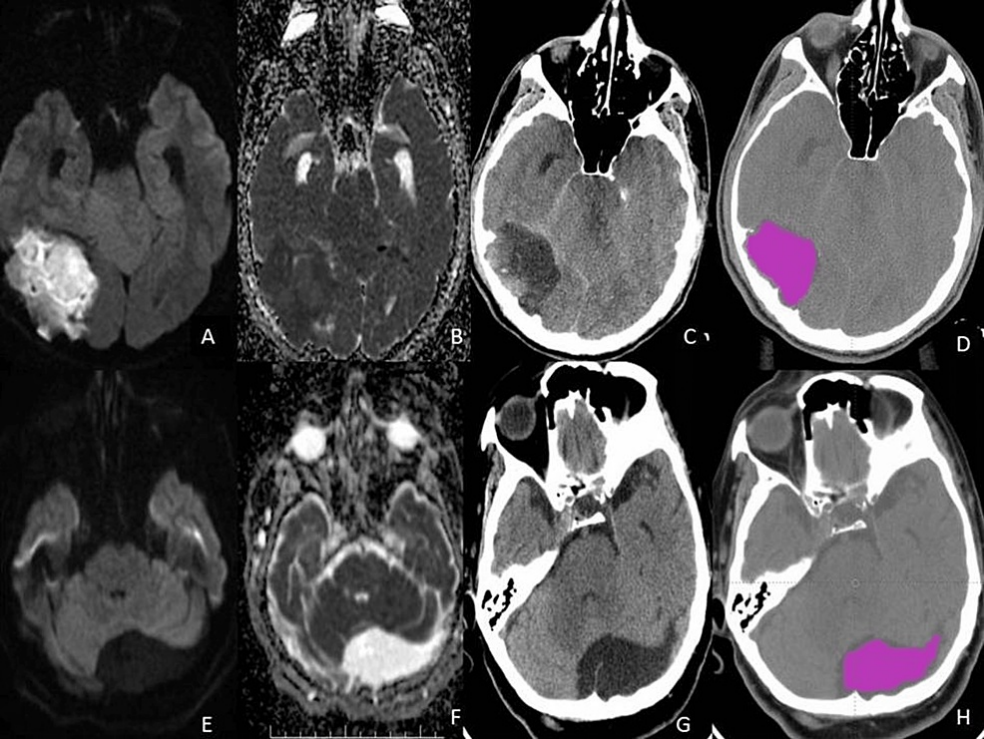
(A): Diffusion-weighted imaging (DWI) of the epidermoid cyst; (B): Apparent diffusion coefficient (ADC) of the epidermoid cyst; (C): Computed tomography (CT) of the epidermoid cyst; (D): Segmentation of the epidermoid cyst; (E): DWI of the arachnoid cyst; (F): ADC of the arachnoid cyst; (G): CT of the arachnoid cyst; (H): Segmentation of the arachnoid cyst
